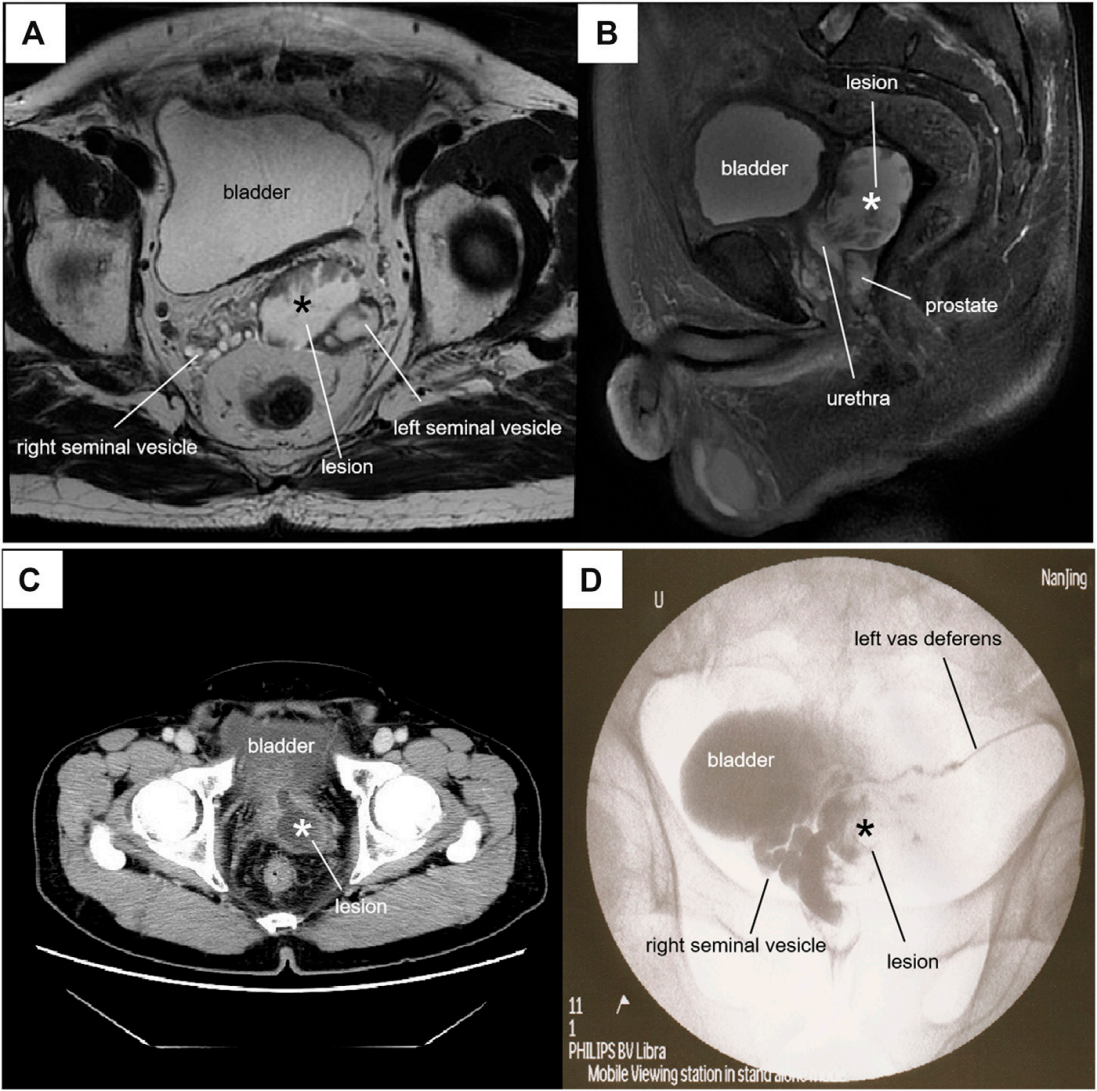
Radiographic features of the lesion*. (A) Axial T2-weighted MRI showed a 45 × 36×44-mm lesion with mixed signals at the left lower posterior of the bladder. (B) Sagittal fat-suppressed T2-weighted MRI showed that the cystic-solid lesion passed through the prostate into the posterior urethra. (C) Contrast-CT showed a cystic-solid mass with obvious enhancement of the cyst wall communicating with the urethra. (D) Vasography showed a filling defectan in the cystic structure.
